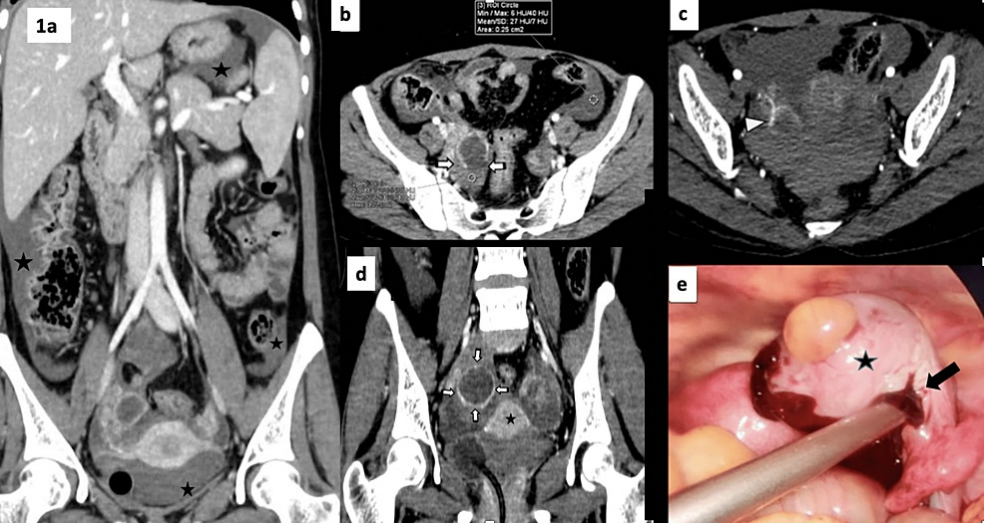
CT and diagnostic laparoscopy images. Contrast-enhanced CT of the lower abdomen showing gross hemoperitoneum in all four quadrants around perihepatic, perisplenic, epigastrium, and pelvic regions in coronal reformated images (a). On axial sections (b, c), a rim-enhancing hypodense cystic lesion was seen in the right adnexa with a focal discontinuity in the posterior wall at 4 to 6 o’clock positions (white arrows, b), indicating a ruptured cyst (direct sign) with high attenuation fluid (HU ~51), suggesting a sentinel clot near the ruptured site representing acute clotted hemorrhage (white arrowhead, b, c). Coronal reformatted images showing irregular thick wall enhancement (d) and CT “ring of fire appearance,” suggesting impending rupture or a tensile cyst (arrow, d). Myometrial enhancement was used as a reference (d, star). Diagnostic laparoscopy images (e) showing a large corpus luteal cyst (star) with a cauterized rent and a drain placed (arrow).
